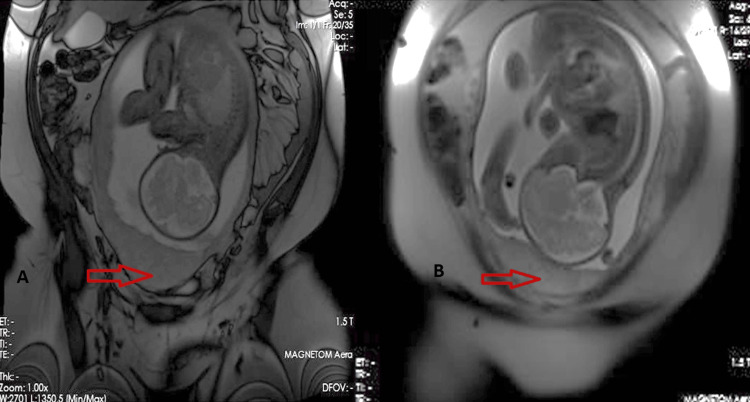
(A) and (B): MRI showing the placenta (red arrows) with dark intraplacental bands
